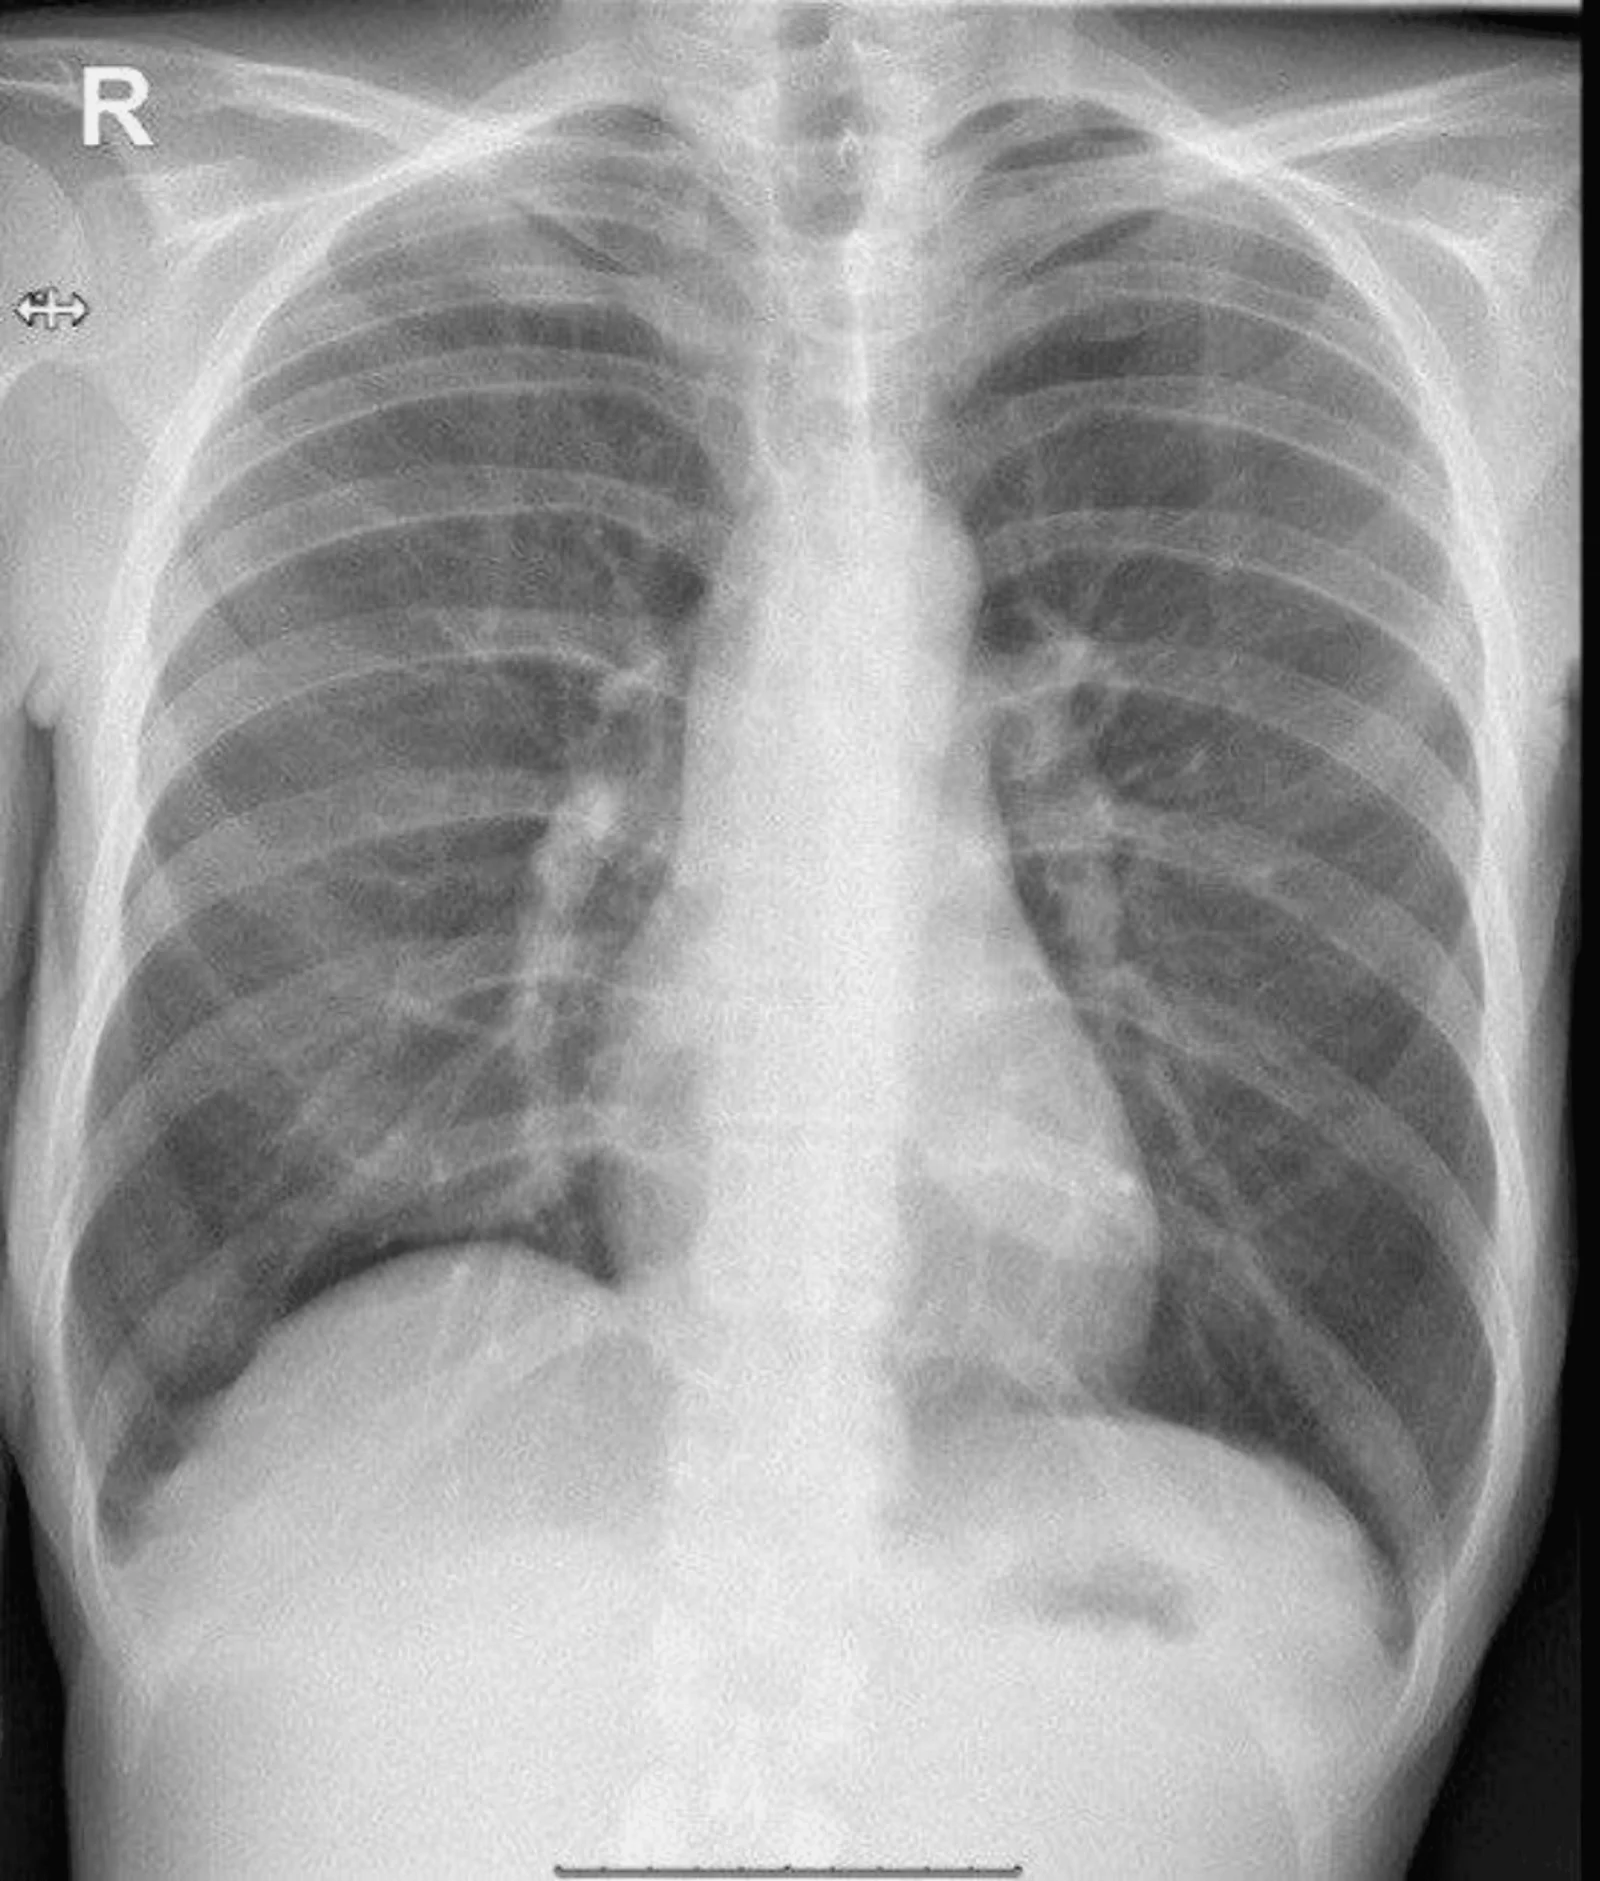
Posteroanterior chest radiograph (Day 1) with radiology report. No focal consolidation, normal cardiac silhouette, clear costophrenic angles, no pneumothorax. Normal chest radiograph.
